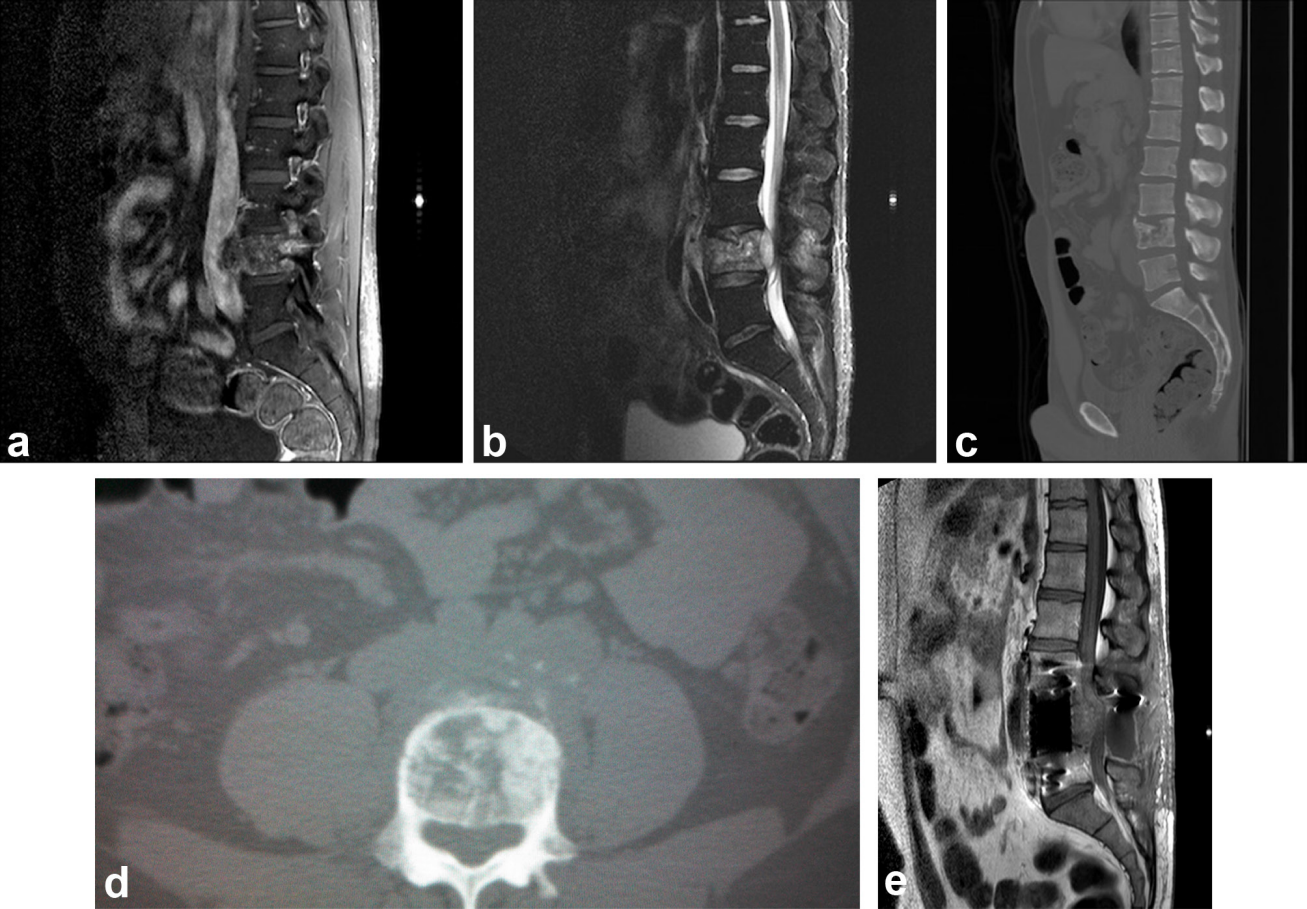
MRI and CT of mesenchymal chondrosarcoma in vertebral body. (a) Sagittal
